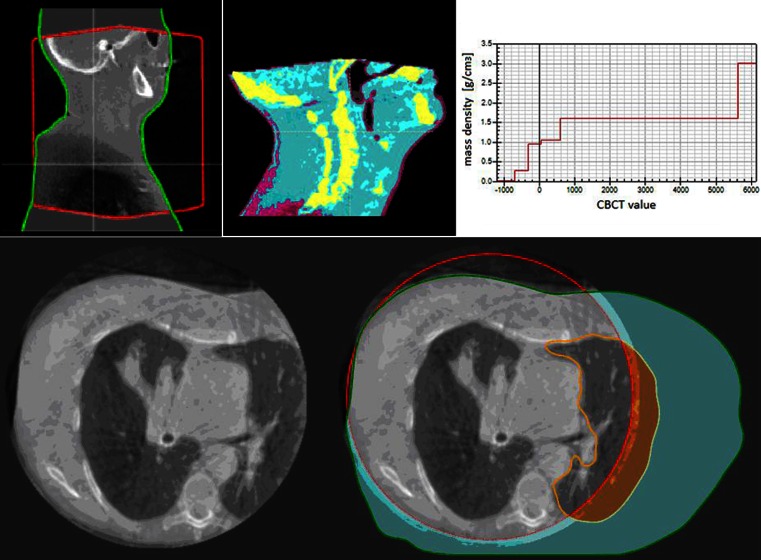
The RayStation treatment planning system (TPS) cone beam computed tomography (CBCT) CT number (CTN) adjustment method. *Top row from left*: Sagittal slice of a CBCT image of an H&N cancer patient viewed within the TPS; the CBCT after density assignment by the TPS (regions assigned as bone are shown as *yellow*, for example); CTN-density table generated for the CBCT image. *Bottom row, left:* Typical CBCT acquisition of a patient with a tumor in their right lung and (*right*) the same CBCT image but with the field of view (*red contour*), external (*green contour*), and left lung (*orange contour*) regions of interest displayed
